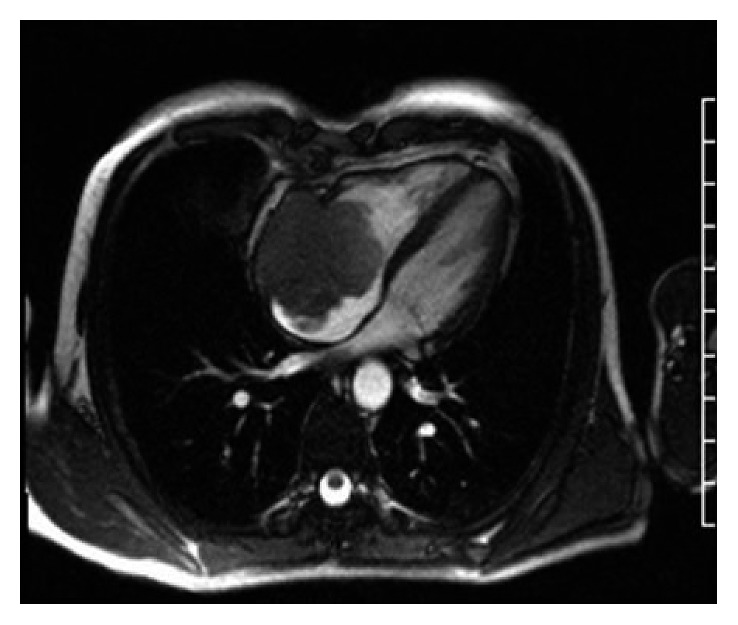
Cardiac MRI demonstrating 5.3 × 5.4 right atrial mass.
